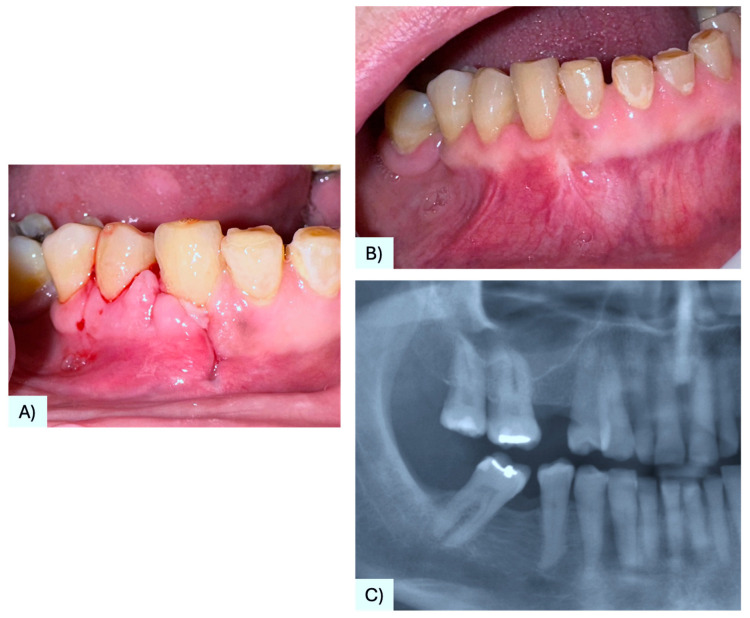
Partial healing of the treated area at 3 weeks clinical follow-up (A); complete clinical healing of the soft tissue at 2 months follow-up (B); 3 months radiographic follow-up showing no bone alteration, also with healing of the treated site (C).
